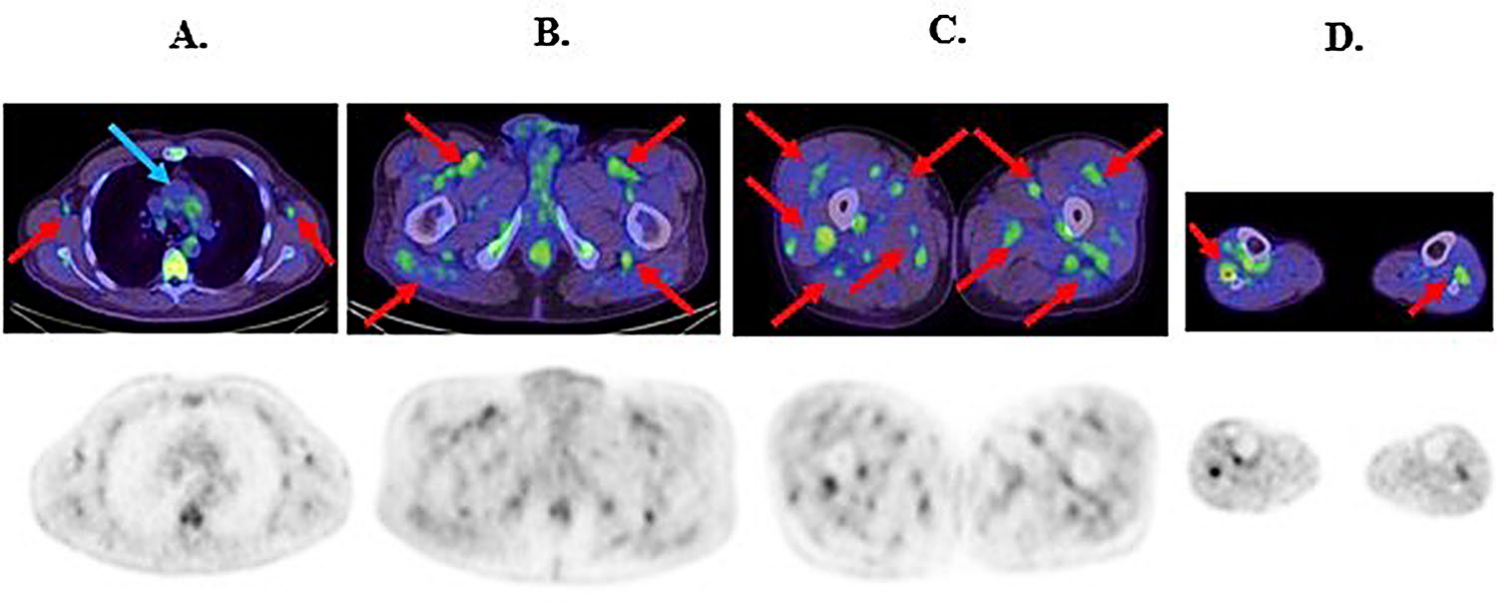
A ^18^F-FDG PET/CT trans-axial fused and ^18^F-FDG PET images at the level of the axilla demonstrating vasculitis of bilateral axillary vessels (red arrows). No abnormal ^18^F-FDG uptake is demonstrated in the ascending aorta (blue arrow). B ^18^F-FDG PET/CT trans-axial fused and ^18^F-FDG PET images at the level of the proximal thighs demonstrating vasculitis of bilateral femoral vessels and multiple medium sized gluteal vessels (red arrows). C ^18^F-FDG PET/CT trans-axial fused and ^18^F-FDG PET images at the level of the mid thighs demonstrating vasculitis of bilateral popliteal vessels and multiple other medium sized vessels (red arrows). D ^18^F-FDG PET/CT trans-axial fused and ^18^F-FDG PET images at the level of the proximal calves demonstrating vasculitis in multiple medium sized vessels (red arrows)
